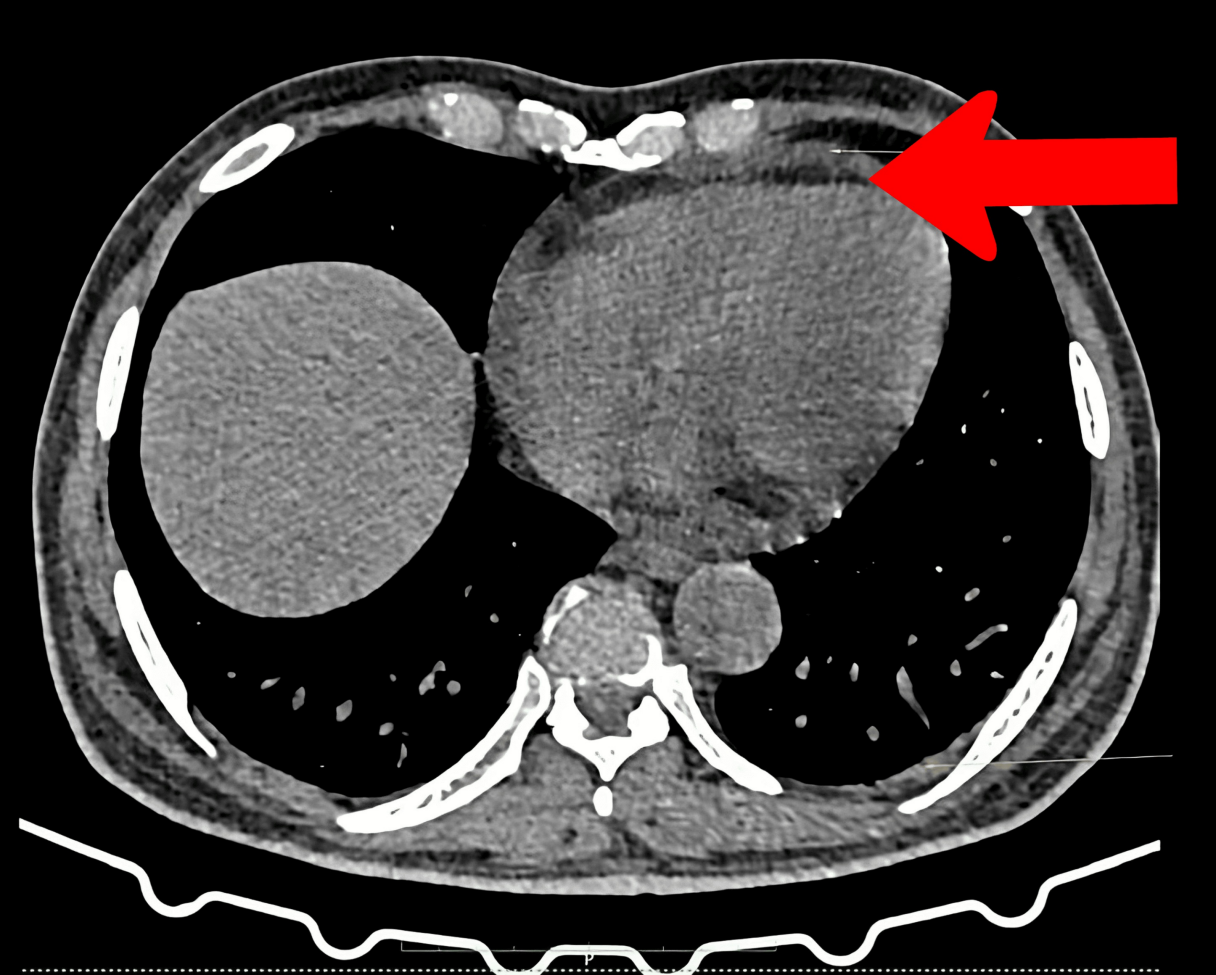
High-resolution CT images of the axial section of the thorax Red arrow shows mild pericardial effusion.
